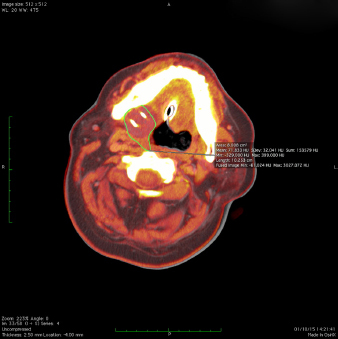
Fusion of the preablation magnetic resonance imaging (MRI) and the ablation computed tomography (CT) volume during cryoablation. Three different positions of cryoprobes and ice balls encompassing the MRI visible tumour volume (green line).
